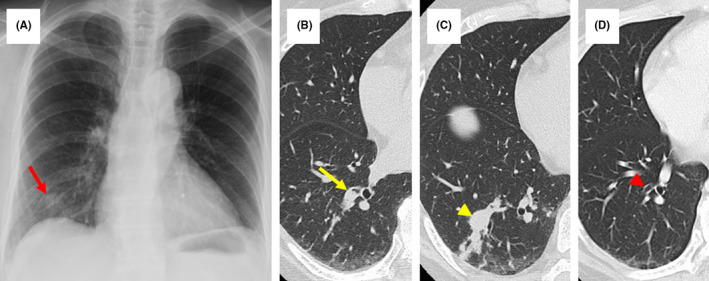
Imaging findings. Chest X‐ray findings showing a 15 × 16‐mm‐sized nodule in the right lower lobe of the lung (A, red arrow). Chest computed tomography findings showing stenosis in the right B9 bronchus (B, yellow arrow) and a 13 × 16‐mm‐sized elliptic nodule with consolidation in the S9 segment of the right lower lobe (C, yellow arrowhead). No visible stenosis in the B9 bronchus at 2 years after the first‐line antituberculosis administration is observed (D, red arrowhead)
